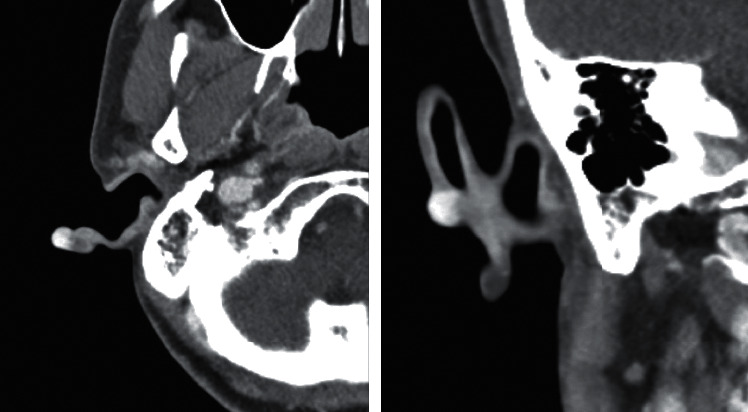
Facial computed tomography revealing a 0.8 × 0.6 cm strongly enhanced mass in the right auricle. (a) Axial image; (b) coronal image.
